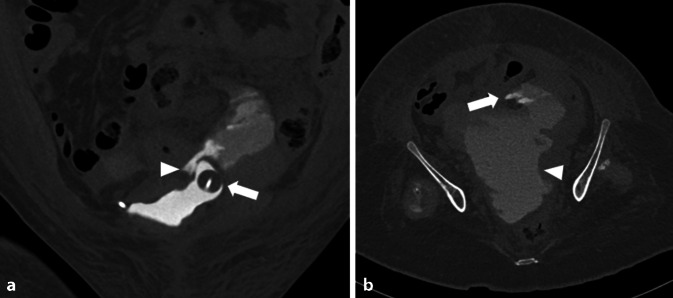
Computertomographie (CT) des Abdomens in urographischer Phase in a koronarer und b axialer Rekonstruktion (Knochenfenster). a Zwischenzeitlich wurde ein Harnblasenkatheter über die Ausleitung im rechten Unterbauch eingebracht. Der geblockte Ballon liegt im Divertikel (*Pfeil*). Zudem Nachweis von austretendem Kontrastmittel/Urin (*Pfeilspitze*). b Abzugrenzen ist das austretende Kontrastmittel/Urin mit Ausbildung eines großen Urinoms im Unterbauch (*Pfeilspitze*)
